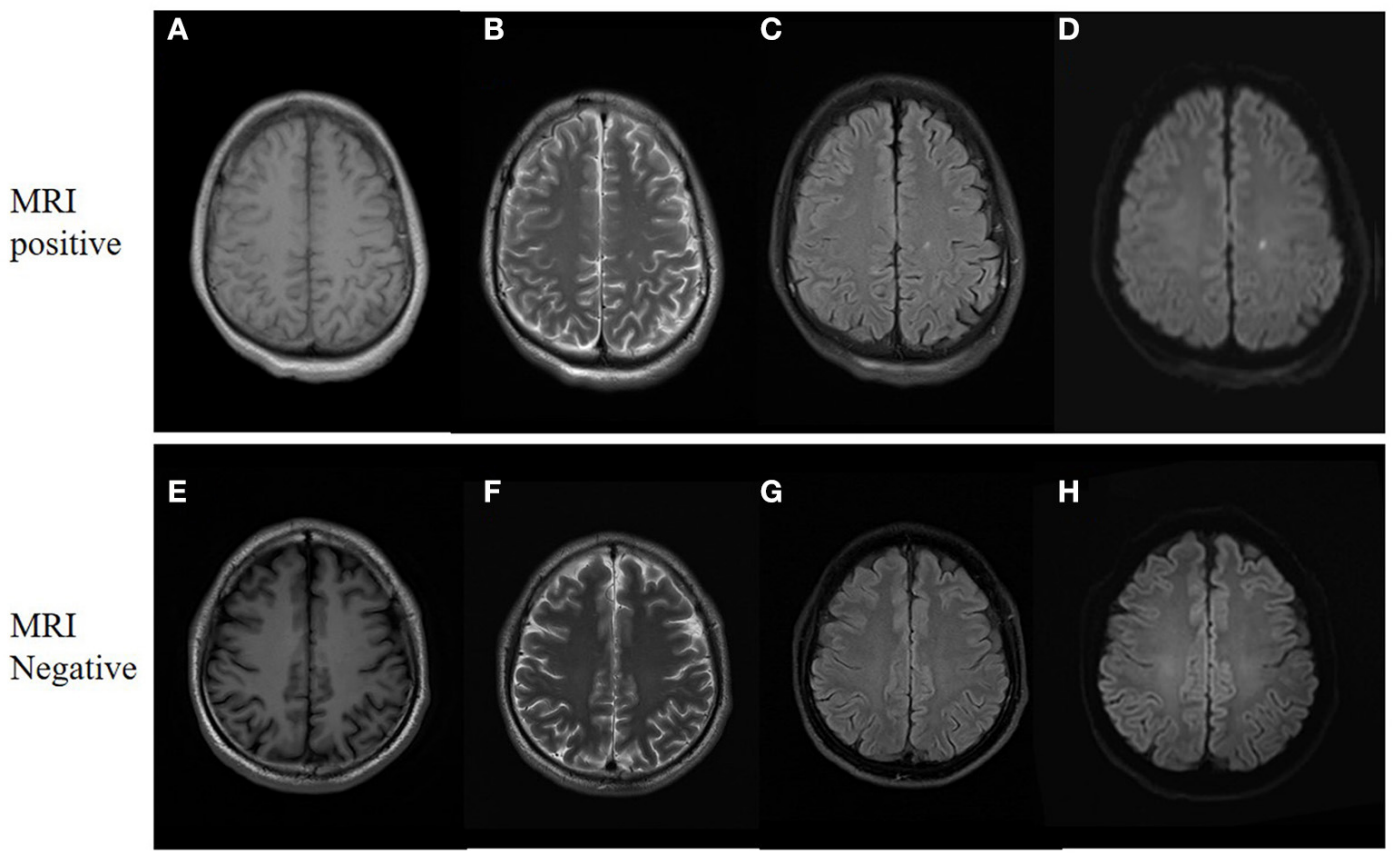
MRI manifestations of two mTBI patients. (A–D), a 31-year-old male with mTBI. Axial non-contrast MRI shows a small punctate contusion of the left corona radiata. The ALPS index is 1.45. (E–H), a 33-year-old male with mTBI. The MRI findings were negative, whereas the ALPS index is 1.46. (A, E) are T1 weighted images. (B, F) are T2 weighted images. (C, G) are T2-Flair images. (D, H) are diffusion weighted images.
